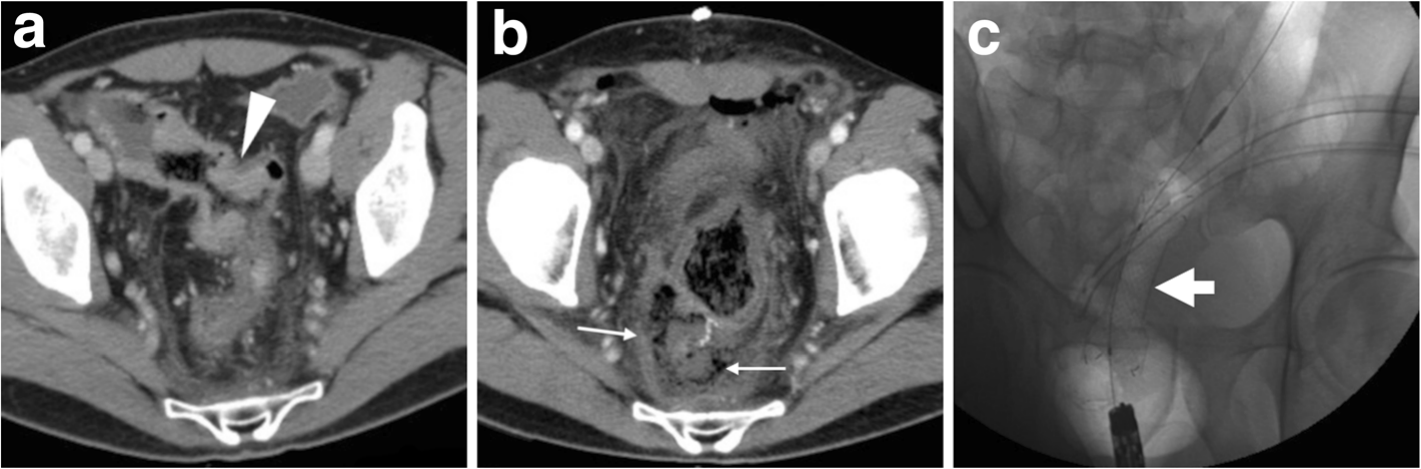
Successful stent management of anastomotic leak (AL). After the CT diagnosis of CRC at the distal sigmoid colon (arrowhead in a), an elderly male underwent surgical resection with primary anastomosis. Early postoperative CT (b) showed inhomogeneous collection (thin arrows) containing gas bubbles, located between the anterior aspect of the sacrum and the colorectal anastomosis site, consistent with clinical suspicion of AL. The patient ultimately recovered after having a SEMS (thick arrow in c) placed through the anastomosis for 3 months
